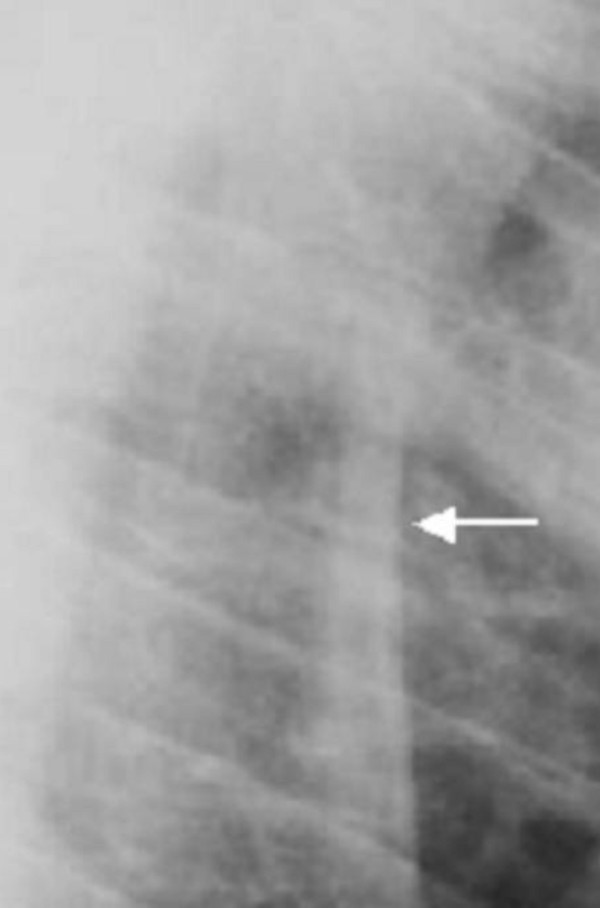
(patient 2). Lateral radiograph of the thoracic vertebrae showed progressive sclerosis of the anterior longitudinal ligaments with subsequent development of bony ankylosis (arrow).
